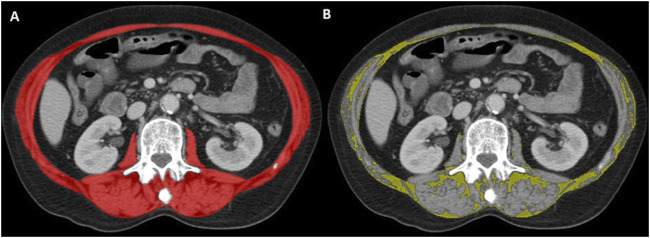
Representative axial CT image at the level of the 3^rd^ lumbar vertebra. Artificial intelligence-based segmentation of abdominal wall and paraspinal muscles in red (A). This surface was used to determine the skeletal muscle area (SMA; red minus yellow surface, not shown) and intermuscular adipose tissue (IMAT) area in yellow (B) according to standard threshold values (−29 to 150 HU for muscle, −190 to −30 HU for fat).
